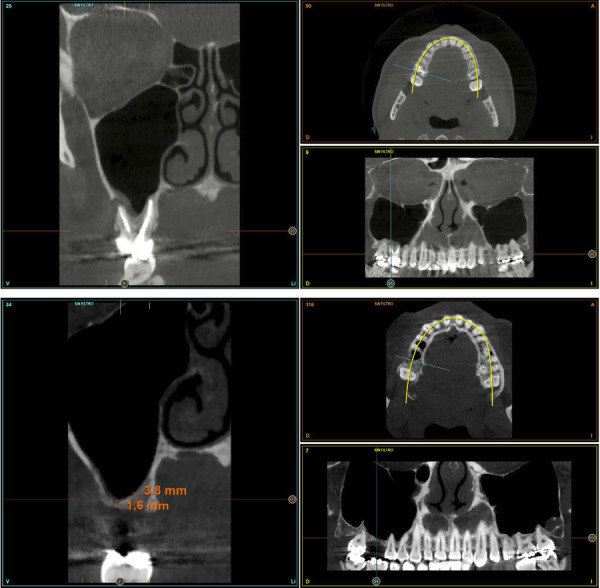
Imagen inicial del *cone-beam* con el molar situado en posición 16 con un quiste apical que precisa ser extraído. En el corte de control tras la exodoncia y regeneración del alveolo con Endoret-PRGF nos muestra una altura de 3,5 mm en la zona de mayor volumen (área palatina).
